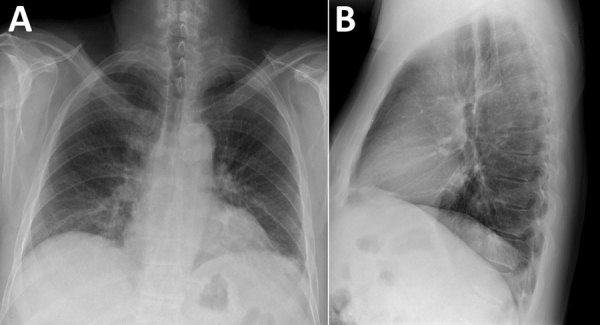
Chest radiographs displaying bibasilar patchy interstitial opacities in patient with hantavirus pulmonary syndrome, Colorado, USA. A) Posteroanterior view. B) Lateral view.
